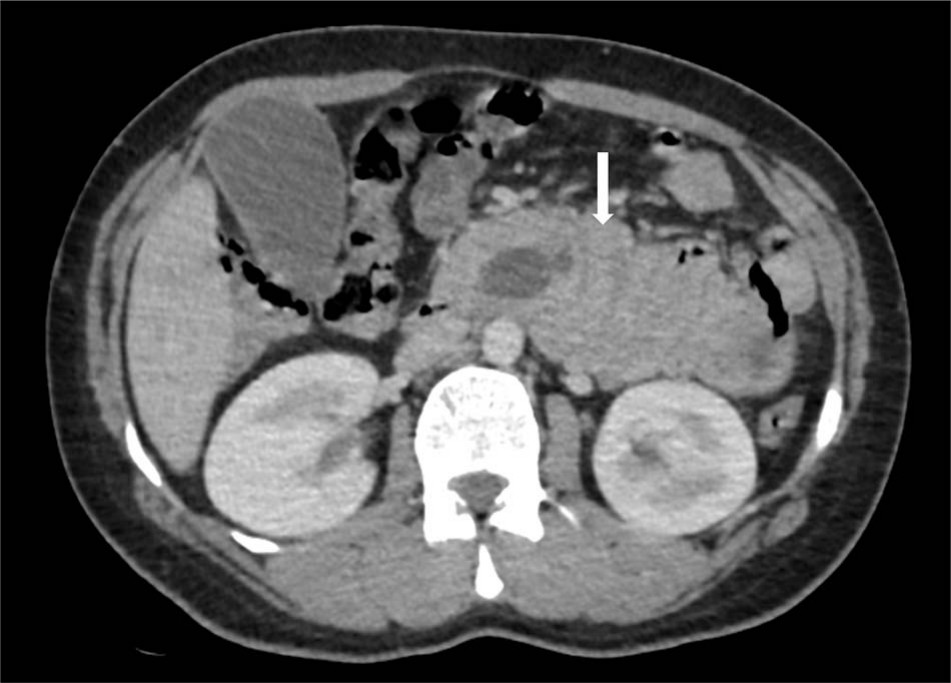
Axial contrast-enhanced CT image showing a duodenojejunal intussusception (arrow), originating from the fourth portion of the duodenum and involving invagination of a proximal jejunal loop.
